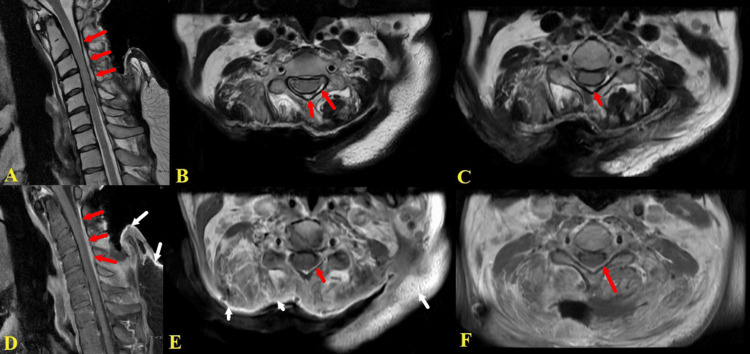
Posterior cervical osteomyelitis and epidural phlegmon T2 (A-C) and post-contrast T1 (D-F) weighted magnetic resonance images revealing posterior cervical epidural collection that enhances with contrast and extends from C2 to T1 (red arrows). Soft tissue signal abnormalities on contrast T1 are also noted, indicating inflammation due to infection (white arrows). Additionally, there is mild compression of the spinal cord caused by the epidural collection. Edges of the wound defect can also be appreciated.
